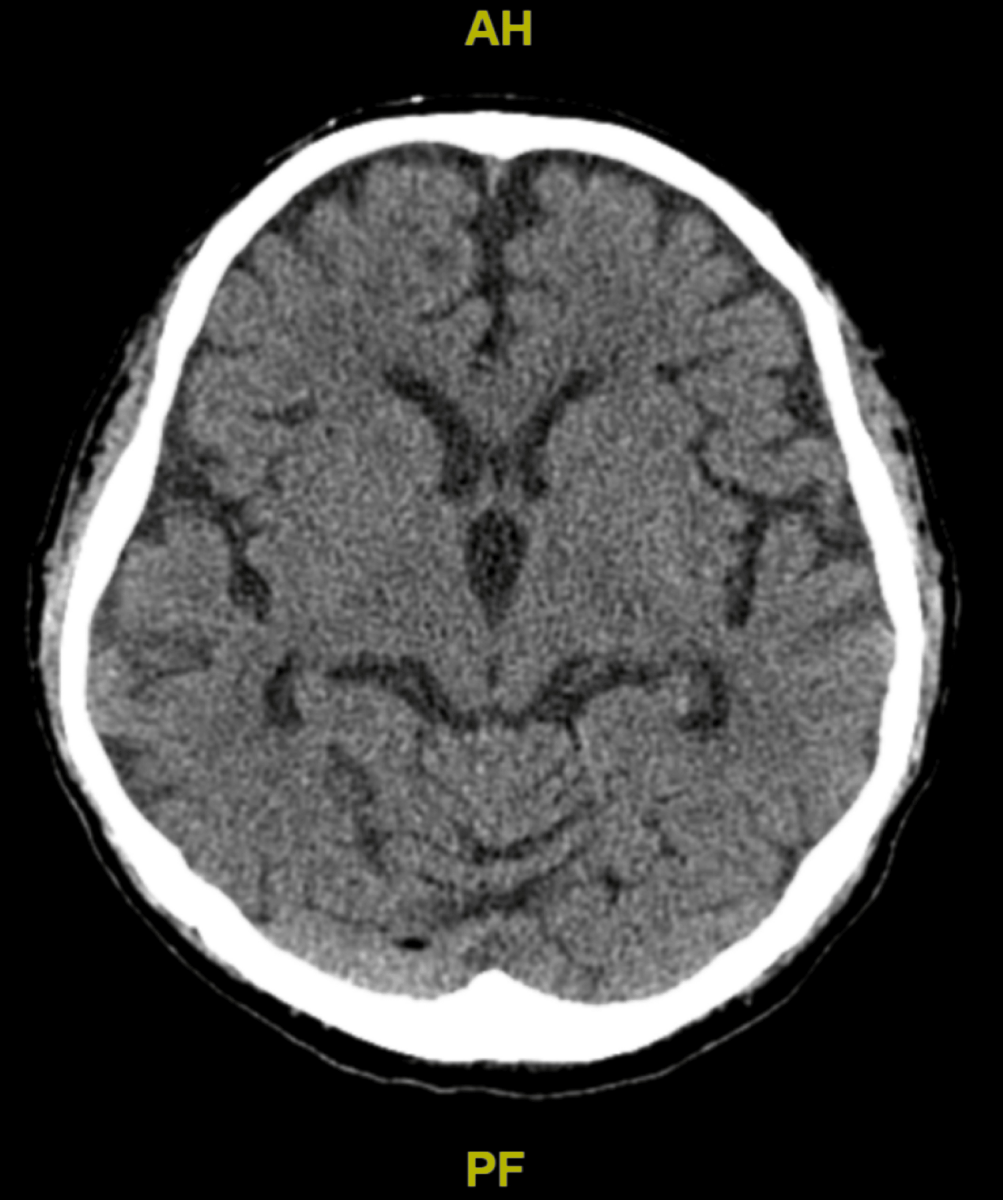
CT head without contrast. Negative for any acute intracranial pathology including signs of inflammation, stroke, hemorrhage, masses or midline shift.
